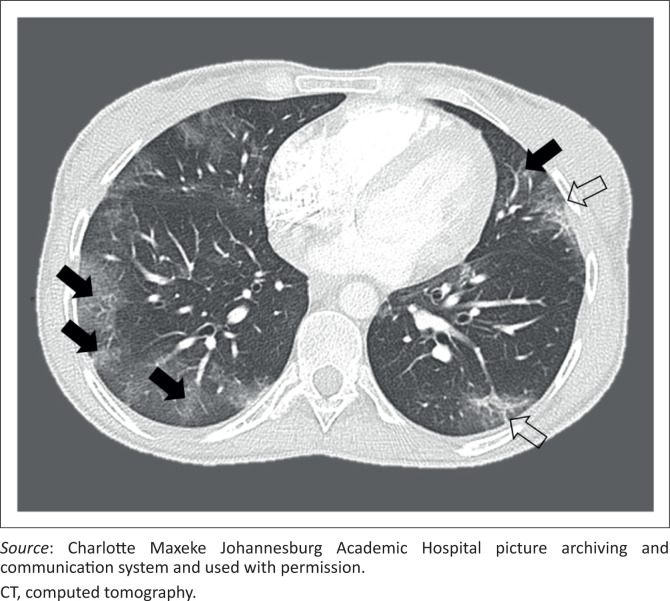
Axial CT lung window of a 31-year-old female patient with no comorbidities, who presented with dyspnoea, fever and a sore throat. Typical findings of peripheral patchy ground glass opacities in the lower lobes (black arrows) with interlobular septal thickening (open arrows).
